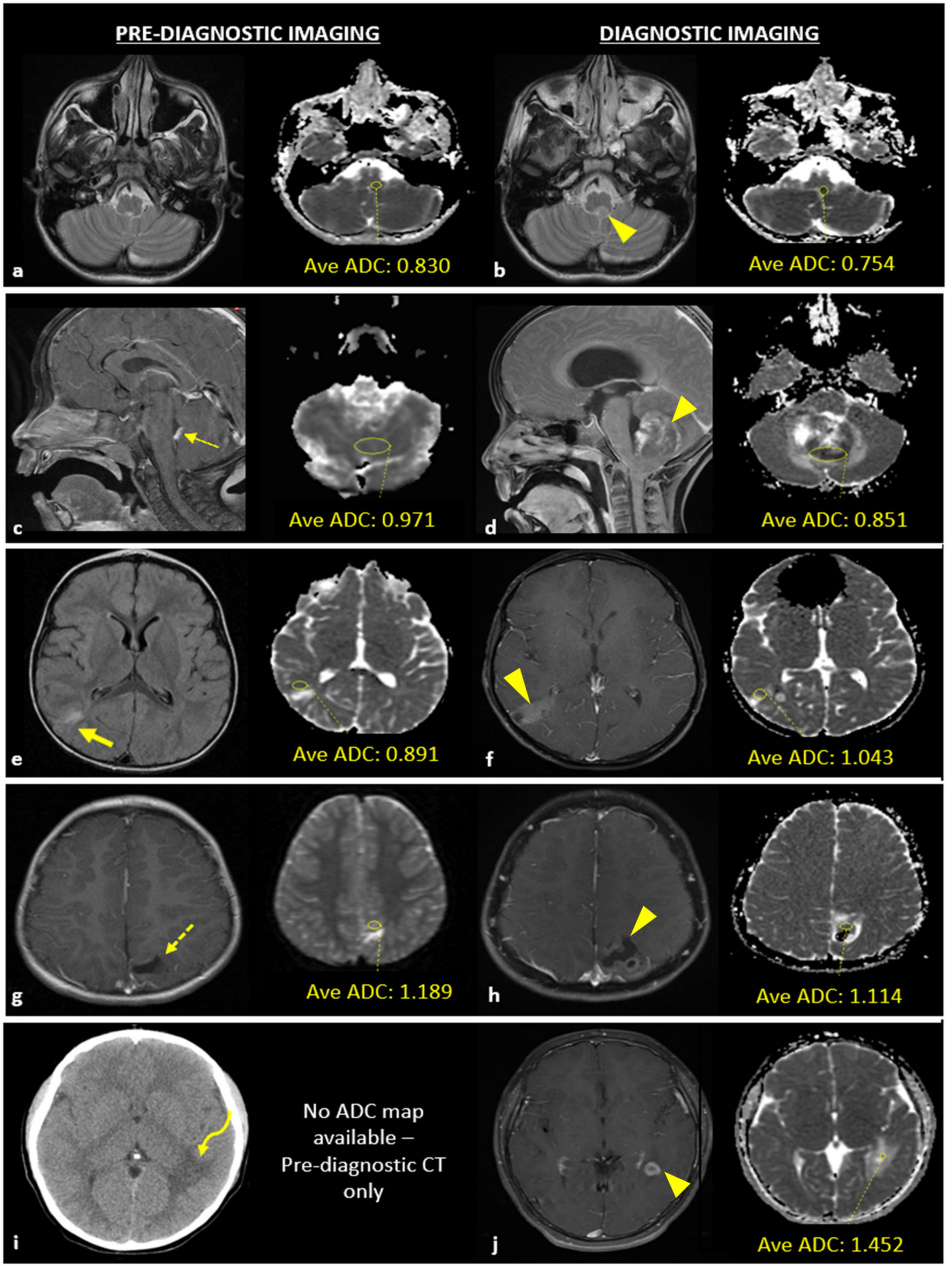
Example of apparent diffusion coefficient (ADC) acquisition for a patient with a case of central nervous system lymphoma (A, B) and examples of neuroimaging studies with abnormal findings at the site of pediatric brain tumor development (C–J). (A) T_2_-W MRI sequence of pre-diagnostic neuroimaging without a macroscopic tumor present and corresponding ADC map with a yellow circle that delineates the area used to calculate average ADC value of the tissue region in which a brain tumor would later develop; (B) T_2_-W MRI sequence obtained at the time of diagnosis demonstrating the macroscopic lesion (arrowhead) and corresponding ADC map with a yellow circle that delineates the area of the tumor used to calculate average ADC value. (C) Pre-diagnostic contrast enhanced (CE) -T_1_WI sagittal image showing thickened, enhancing choroid (thin arrow) and pre-diagnostic ADC map; (D) post-diagnostic CE-T_1_WI sagittal image and ADC map of atypical teratoid rhabdoid tumor (ATRT). (E) Pre-diagnostic T_2_/FLAIR-WI axial image showing hyperintense T_2_/FLAIR signal (thick arrow) and pre-diagnostic ADC map; (F) post-diagnostic CE -T_1_WI axial image with ADC map of low-grade glioma. (G) Pre-diagnostic CE -T_1_WI axial image showing encephalomalacia/gliosis (dashed arrow) and pre-diagnostic ADC map; (H) post-diagnostic CE -T_1_WI axial image with ADC map of a ganglioglioma. (I) Pre-diagnostic axial CT image showing white matter hypoattenuation (curved arrow); no pre-diagnostic ADC map was available due to lack of MR imaging; (J) post-diagnostic CE-T_1_WI axial image with ADC map of JXG.
